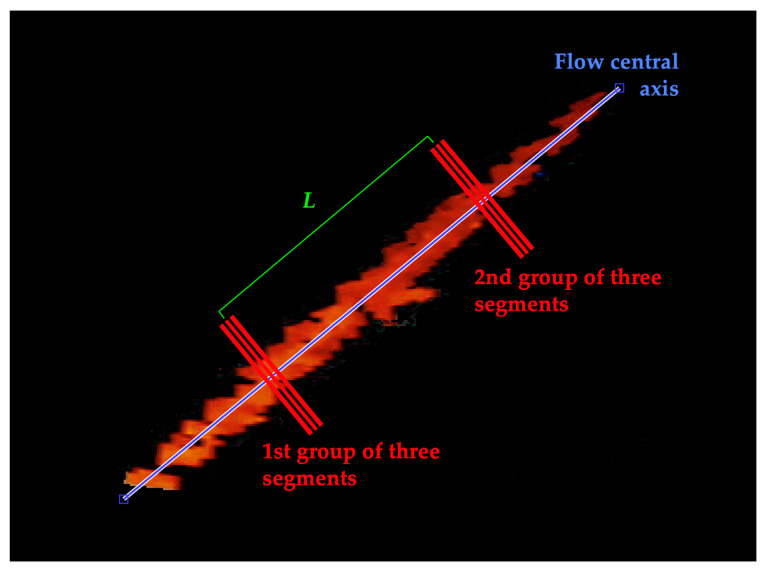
Example of segments positioning on the flow central axis in the first sampled frame. For every group of (three) segments, a sonogram is reconstructed.
